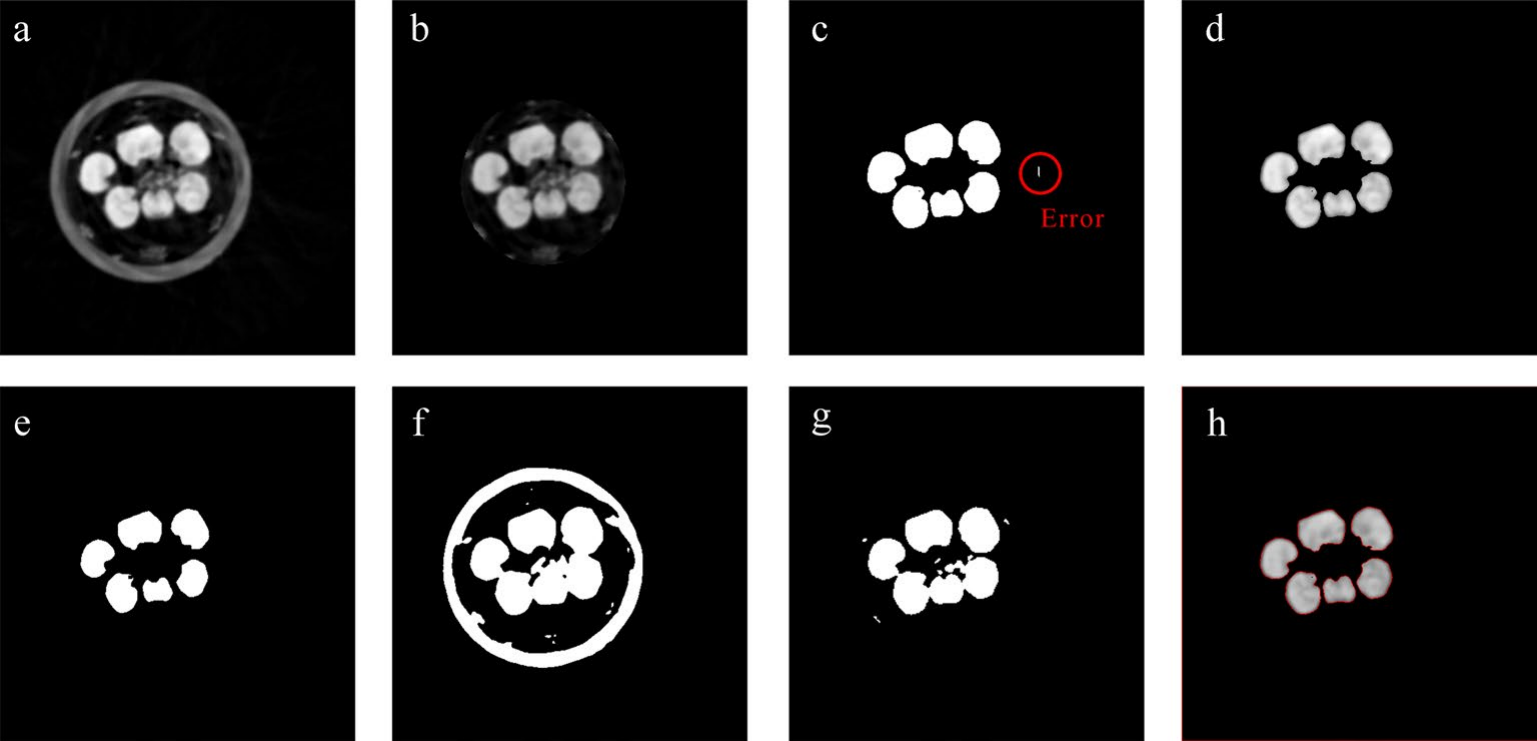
Example of slice image processing for detection of ROI. a Original CT slice image. b Holder-removed slice image. c UNet outputted image prediction of grains. Red circle highlights the error of UNet predicting. d Segmented image. e Assisted threshold segmentation image. f Direct threshold segmentation image without UNet. g Holder-removed direct threshold segmentation image without UNet. h Edge detection image
